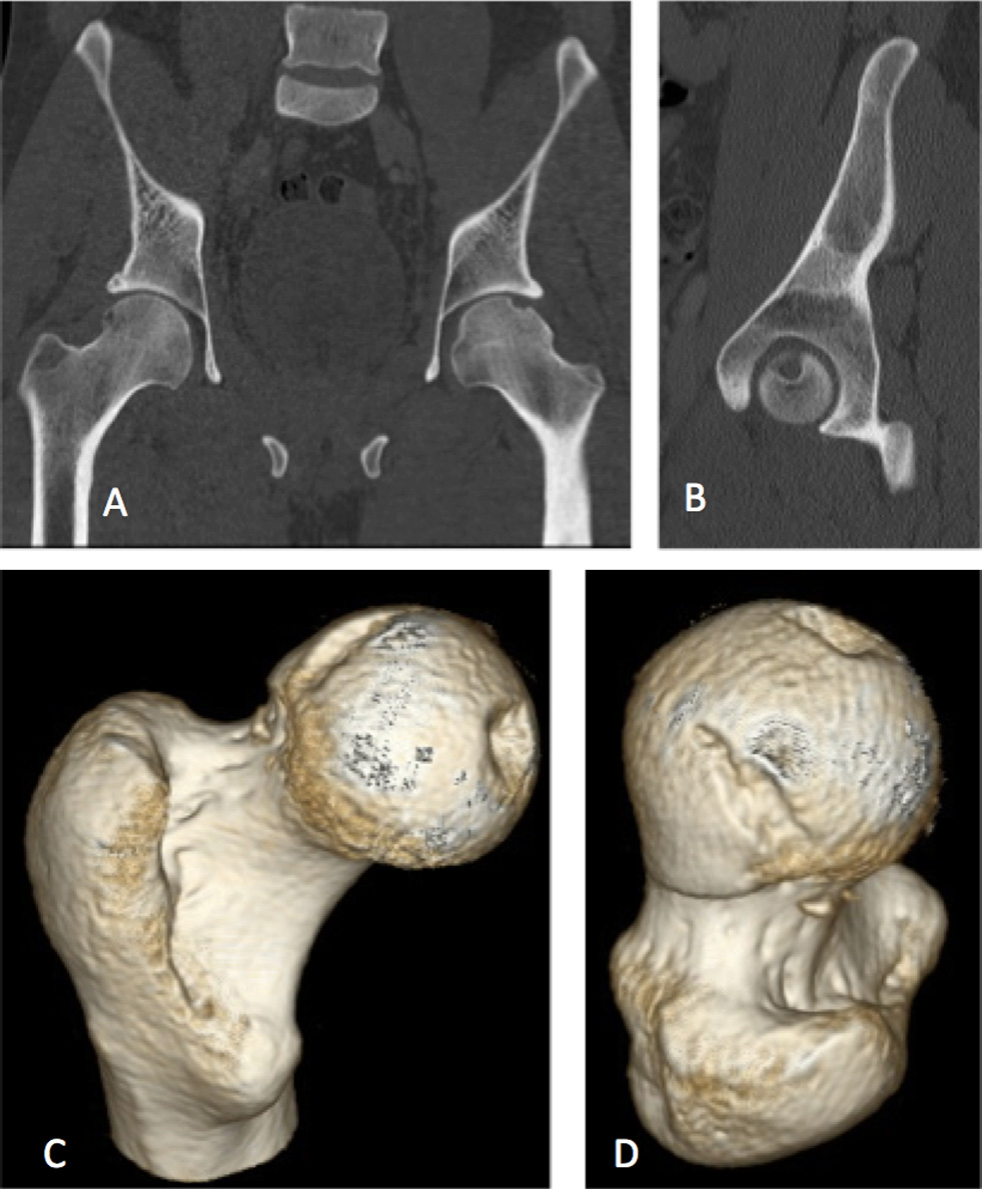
Patient 2: Post Reduction Coronal slice (A), Sagittal slice (B) and 3D reconstruction (C,D) CT demonstrating femoral head impaction and osteochondral defect in weight bearing portion of femoral head.
